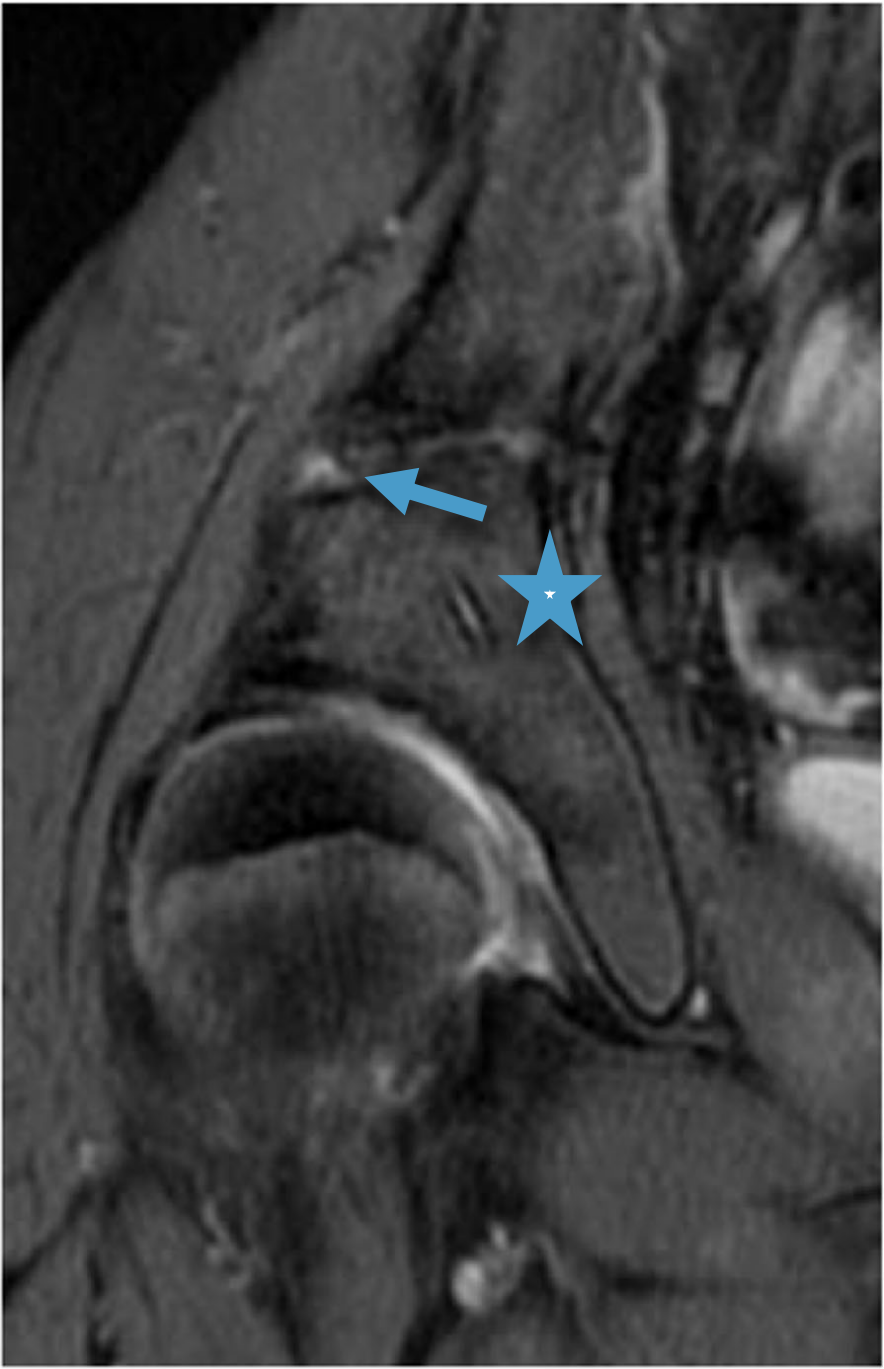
MRI (3T, PD FS/3T) on an 11-year-old girl performed 0.5 years postoperatively after a Triple osteotomy due to hip dysplasia. The screws display mostly fluid-like signal (star), and there is a minor edema (arrow) close to the entry point of one of the screws. The fluid in the screw canal can be traced through the entirety of its length but only a section is visible in the visualized MRI segment
